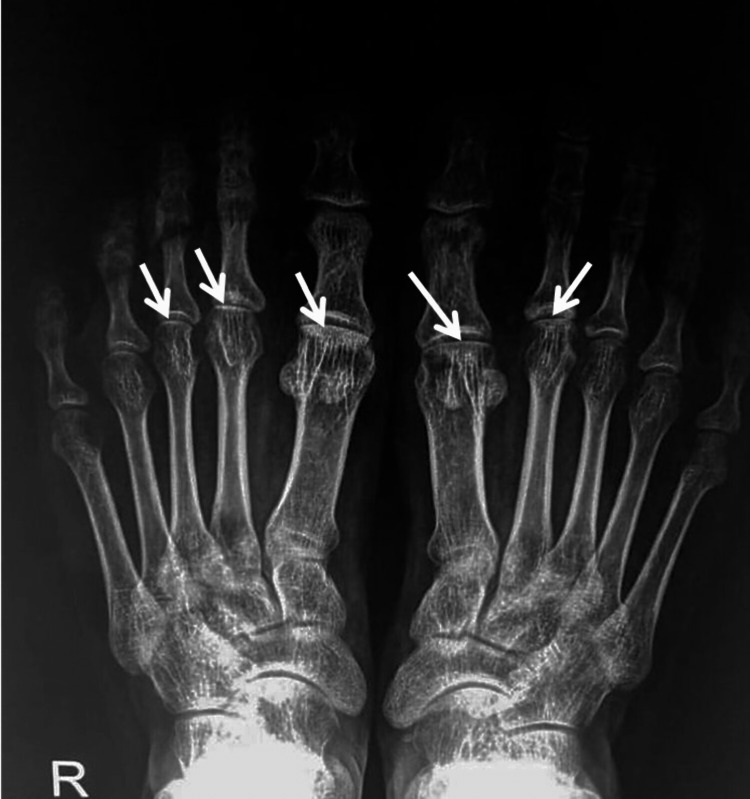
Standing X-rays of feet show flattened and sclerotic changes of the distal ends of metatarsal bones more prominently in the first metatarsal of both feet along with flattening and erosion of the articulation surfaces of the ankle joints bilaterally
